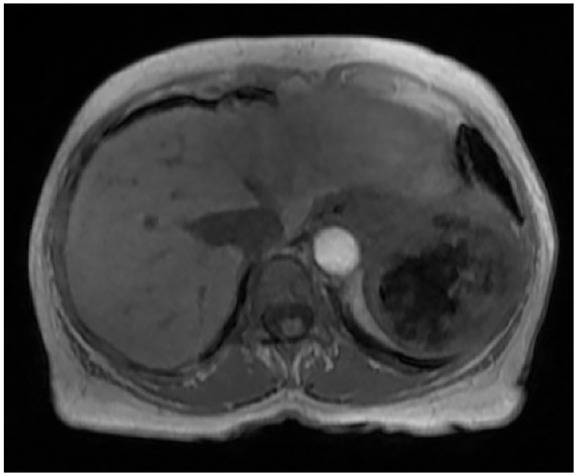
Transaxial* view of abdominal MRI. The presence of 7.9 × 6.2 × 5.7 cm mass with large necrotic center in the spleen.
